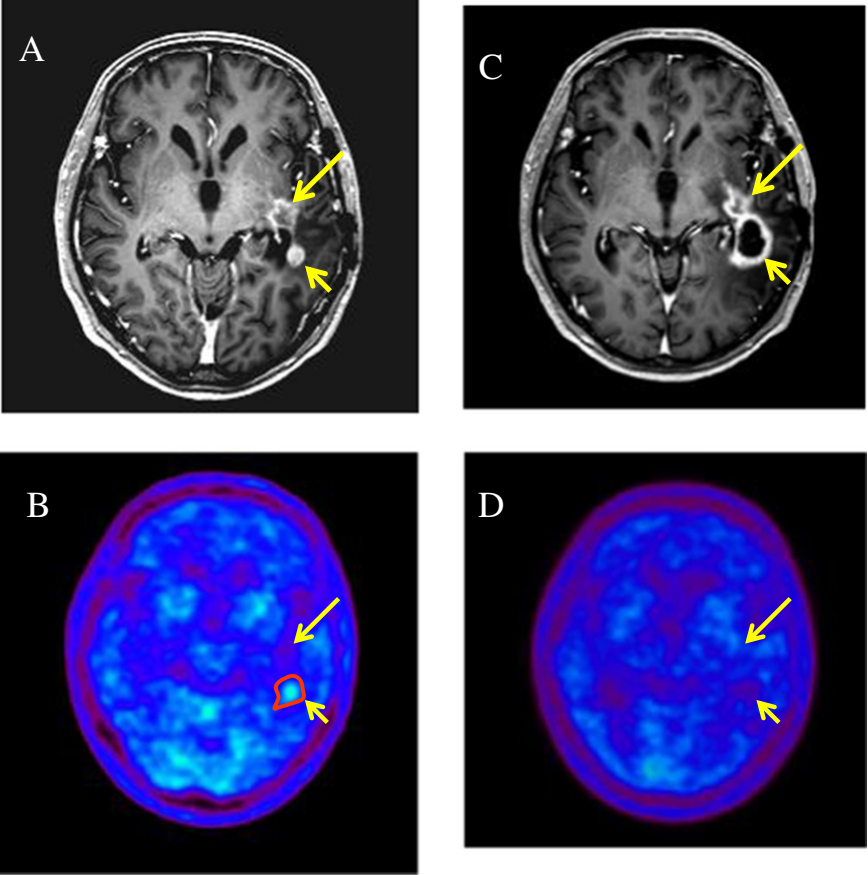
Recurrent glioblastoma multiforme (GBM) in a 55-year-old man before and after hypofractionated stereotactic radiotherapy by intensity modulated radiation therapy (HS-IMRT). Before HS-IMRT, two enhanced lesions (long and short arrow) were demonstrated in the left temporal lobe on T1-weighted magnetic resonance imaging (A). ^11^C-methionine positron emission tomography (MET-PET) demonstrated a MET high-uptake on the region of short arrow, which was defined as the Gross Tumor Volume (red line) (B). 5 months after HS-IMRT, there was no tumor recurrence on the lesion (long arrow, C &D). The enhanced lesion (short arrow) was increased in size (C), although MET uptake decreased relative to normal tissue, which suggested a necrotic change in the irradiated region (D). The patient had no neurologic deficits or quality of life issues. KPS was 90%.
